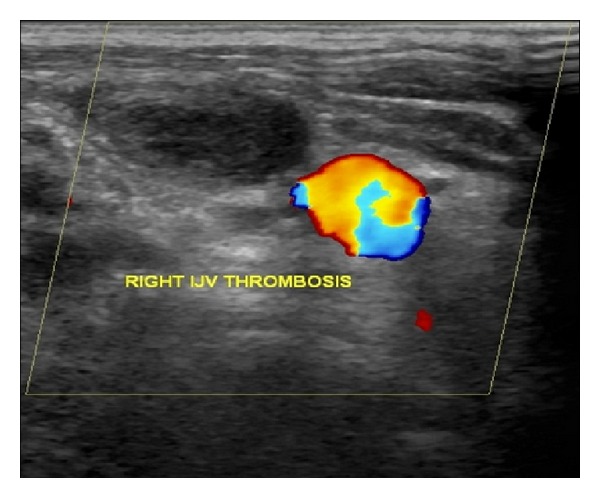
Doppler ultrasonography shows a thrombus in the right internal jugular vein.
